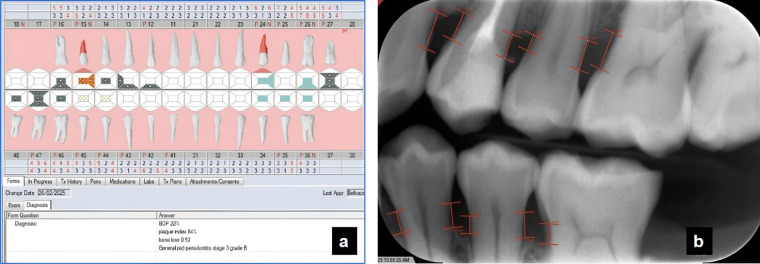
Periodontal Staging and grading done using (a) periodontal examination chart from axium software (b) radiographs labelled from axium software.
